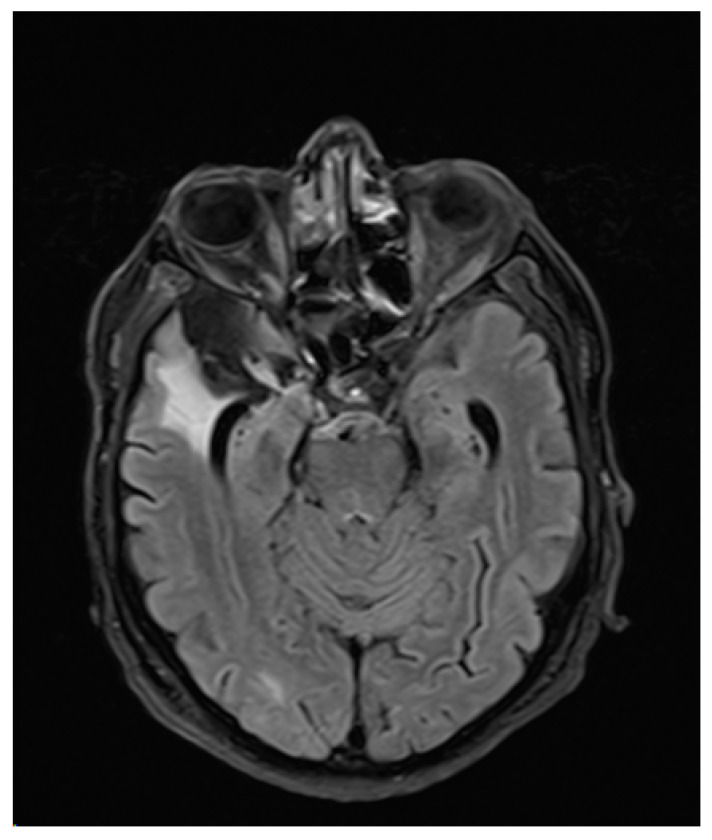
FLAIR cranial MRI image of the parenchymal defect in the right frontobasal region as a result of subarachnoid hemorrhage.
